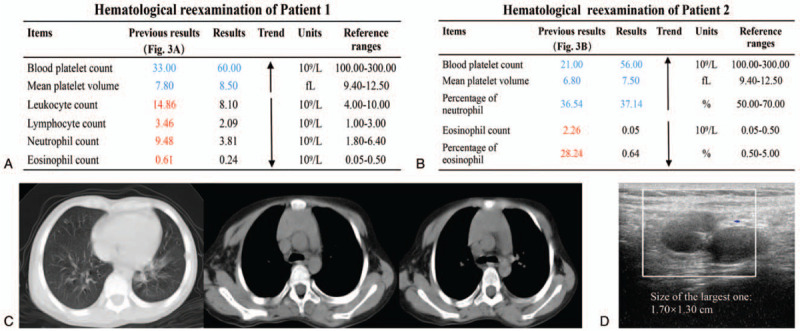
Hematological reexaminations of both patients and imaging reexamination findings of Patient 2. (A) Hematological reexaminations of Patient 1. Compared to the initial examinations (Fig. 3A), the counts of leukocyte, lymphocyte, neutrophil, and eosinophil returned to normal, and the platelet count and mean platelet volume (MPV) showed some recovery. (B) Hematological reexaminations of Patient 2. Compared to the initial examinations (Fig. 3B), the platelet count, MPV, and neutrophil percentage were partially recovered, and the count and percentage of eosinophil returned to normal. In (A) and (B), parameters above and below the reference range were depicted in red and blue, respectively. The upward (downward) arrow indicated an increase (decrease) in the parameter compared to previous results. (C) Image presentation of chest CT of Patient 2. No obvious mediastinal or hilar lymphadenopathy was found, and inflammatory lesions in bilateral lungs were significantly improved than before (Fig. 4F). (D) Abdominal color ultrasound of Patient 2. Multiple mesenteric lymph nodes were detected in the right lower abdomen and around the umbilicus, with the largest one of approximately 1.70 × 1.30 cm, which was significantly smaller than the size at admission (2.30 × 2.00 cm) (Fig. 4G). The white box indicates lymph nodes. CT = computed tomography.
